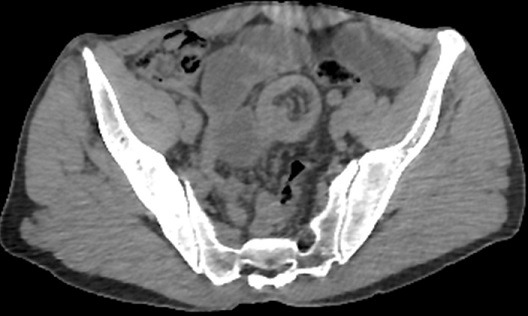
Abdominal non-enhanced helical CT. Transverse axial scan showing the tortuous intestine and its mesentery in the middle low abdomen with a ring-shaped appearance the target sign of the intestinal intussusception
